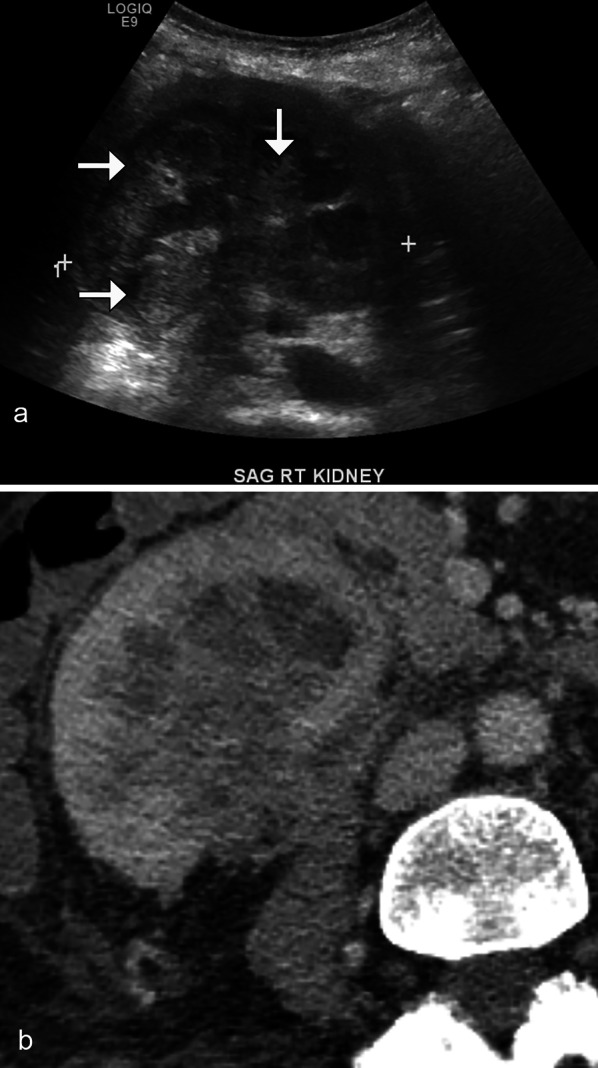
Urothelial carcinoma.* Sagittal grayscale ultrasound (a) demonstrates an enlarged right kidney with relative preservation of the reniform shape. Note the diffuse infiltration of the pelvocalyceal system (arrows). Contrast-enhanced axial CT (b) shows the diffuse enlargement of the right kidney with infiltrative tissue centered in the collecting system; subsequent biopsy confirmed urothelial carcinoma, mimicking the diffuse infiltration/nephromegaly pattern of renal lymphoma
